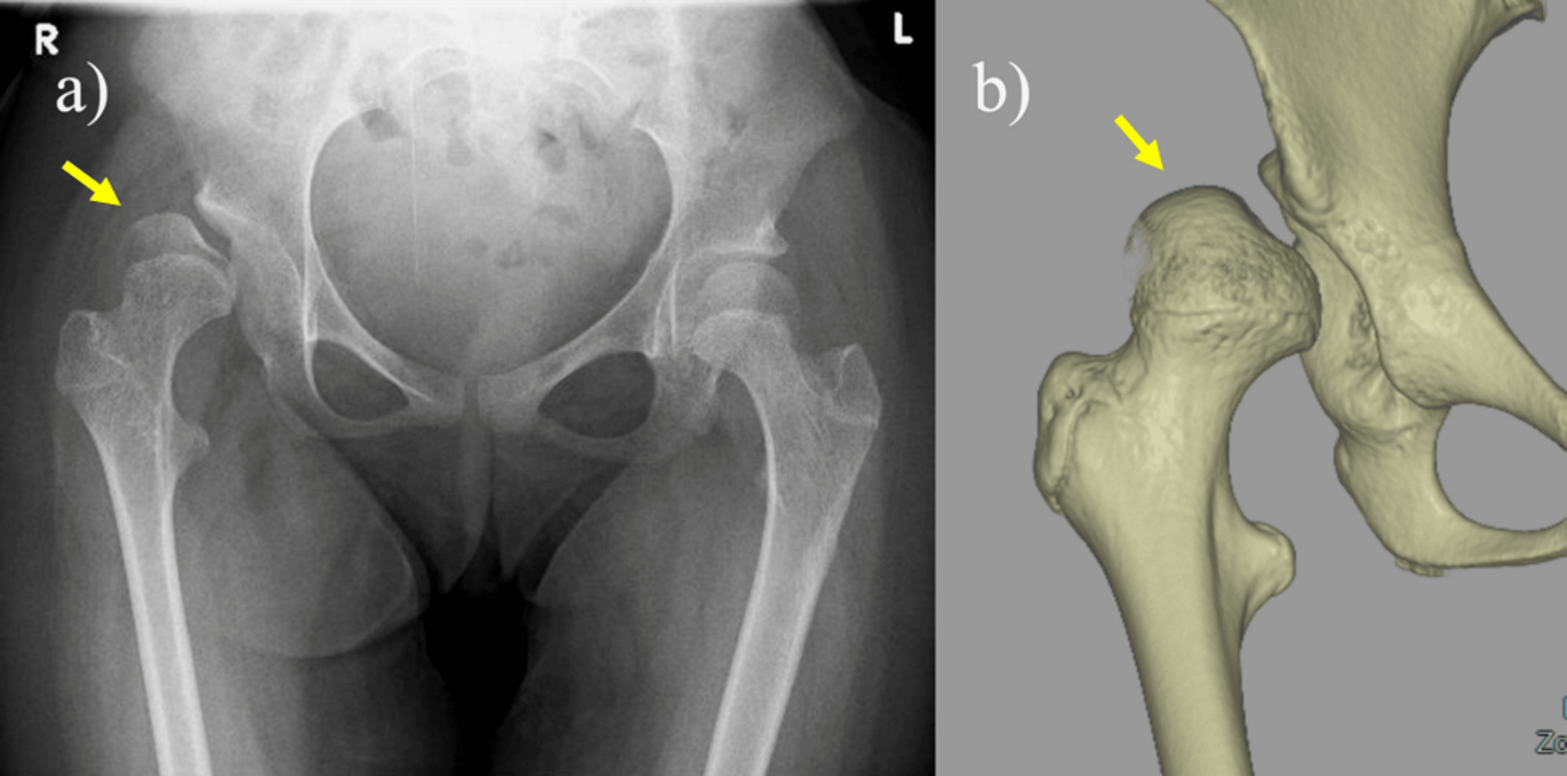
a) Anteroposterior (AP) radiograph and b) a computerized tomography (CT) scan shows a deficient acetabulum and dislocation of the right hip.
